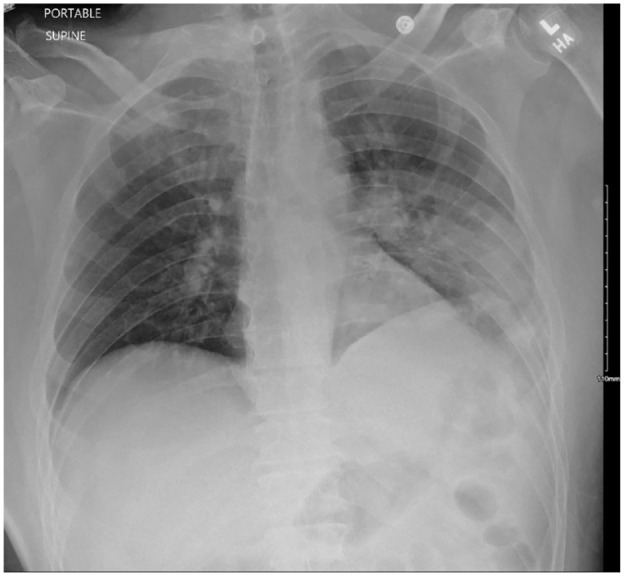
MRI brain: 2 lacunar infarcts seen in right frontal lobe.
